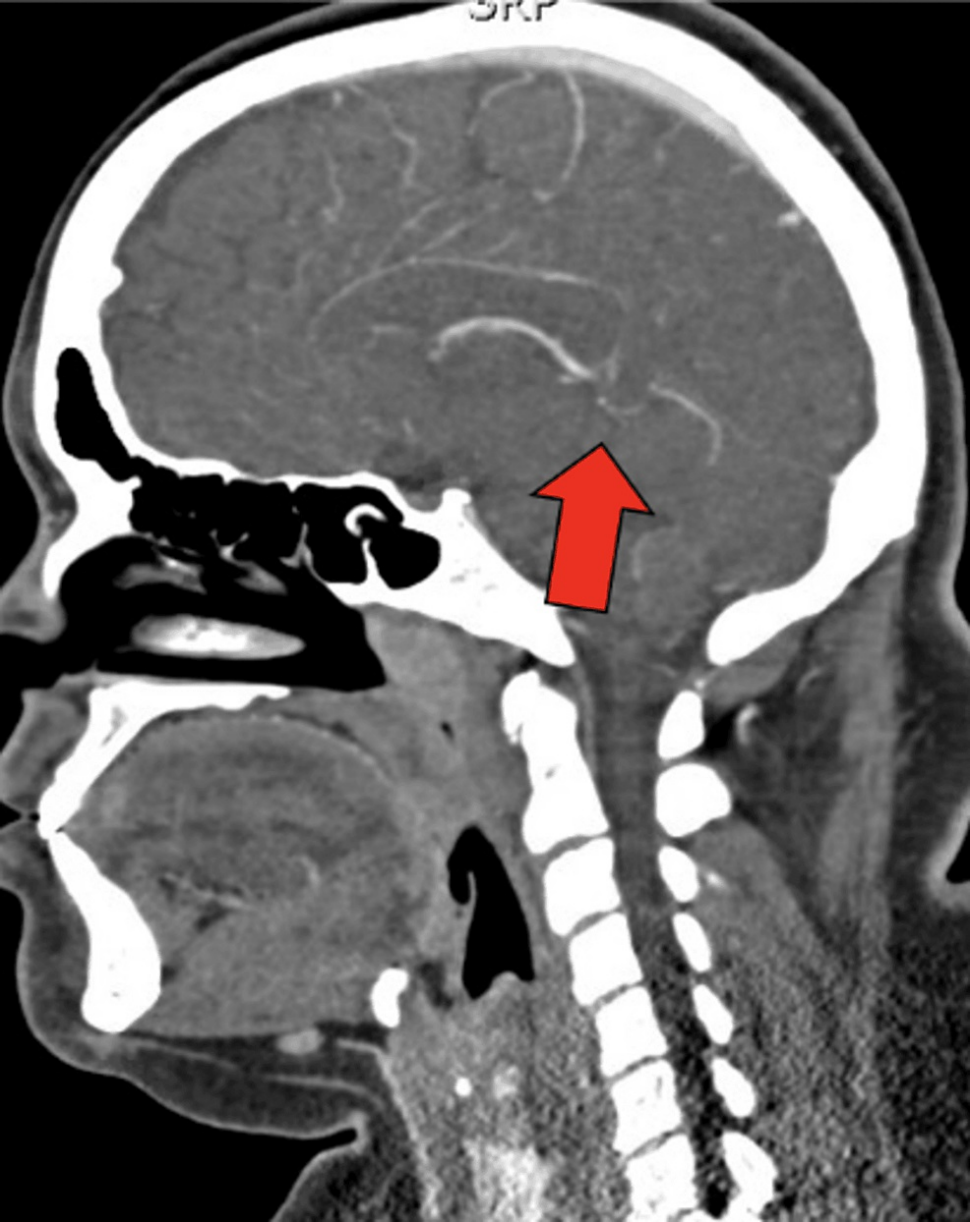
Sagittal CTA image demonstrating right P3 segmental stenosis.
